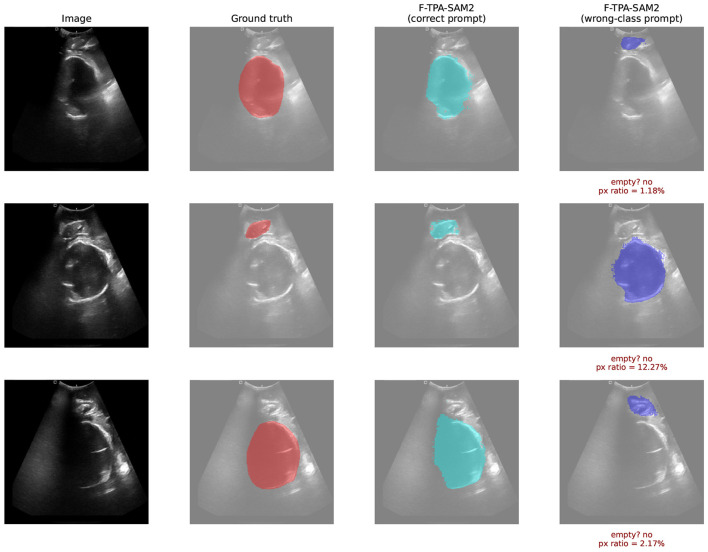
Qualitative PSFHS test examples. Column 3: F-TPA-SAM2 with the correct prompt produces tight masks. Column 4: under a wrong-class prompt, the model segments the *other* structure (consistent with Table 8). The fetal ultrasound images in Figure were adapted from the PSFHS dataset [Lu et al. (23); Zenodo record 10969427; CC BY 4.0].
